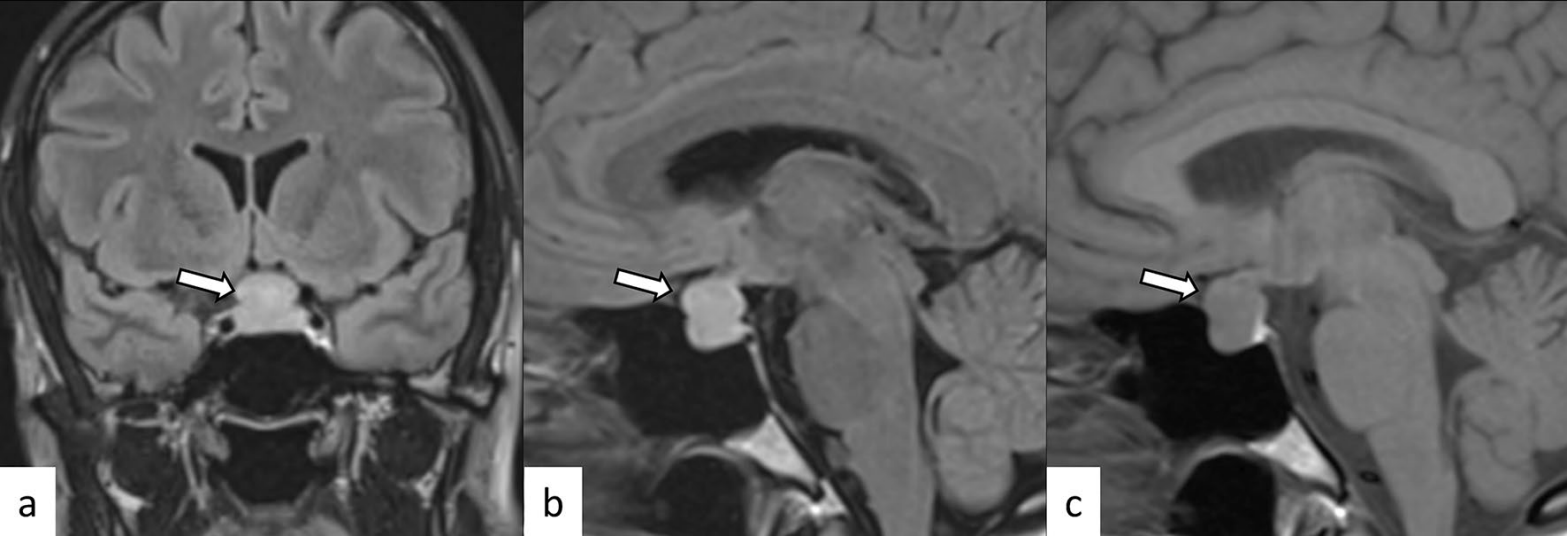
A 37-year-old woman with lymphocytic hypophysitis. A “snowman-like” shaped enlarged pituitary gland is observed (a–c, arrows). The pituitary gland shows homogeneous hyperintensity on fluid-attenuated inversion recovery (FLAIR) coronal and sagittal images (a, b) and homogeneous isointensity on T1-weighted sagittal image (c). The posterior pituitary T1-weighted bright spot is preserved in this case
